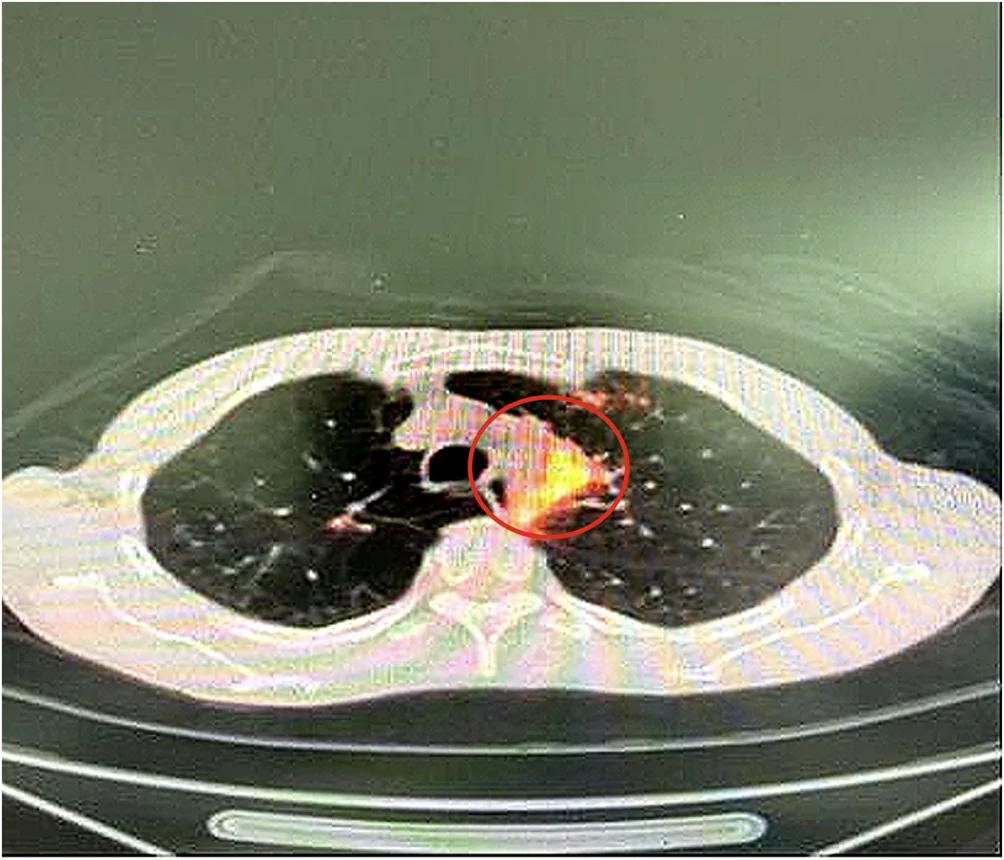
PET‐CT demonstrating intense FDG uptake within the left upper lobe, consistent with a hypermetabolic pulmonary mass (SUVmax: 13.7).
